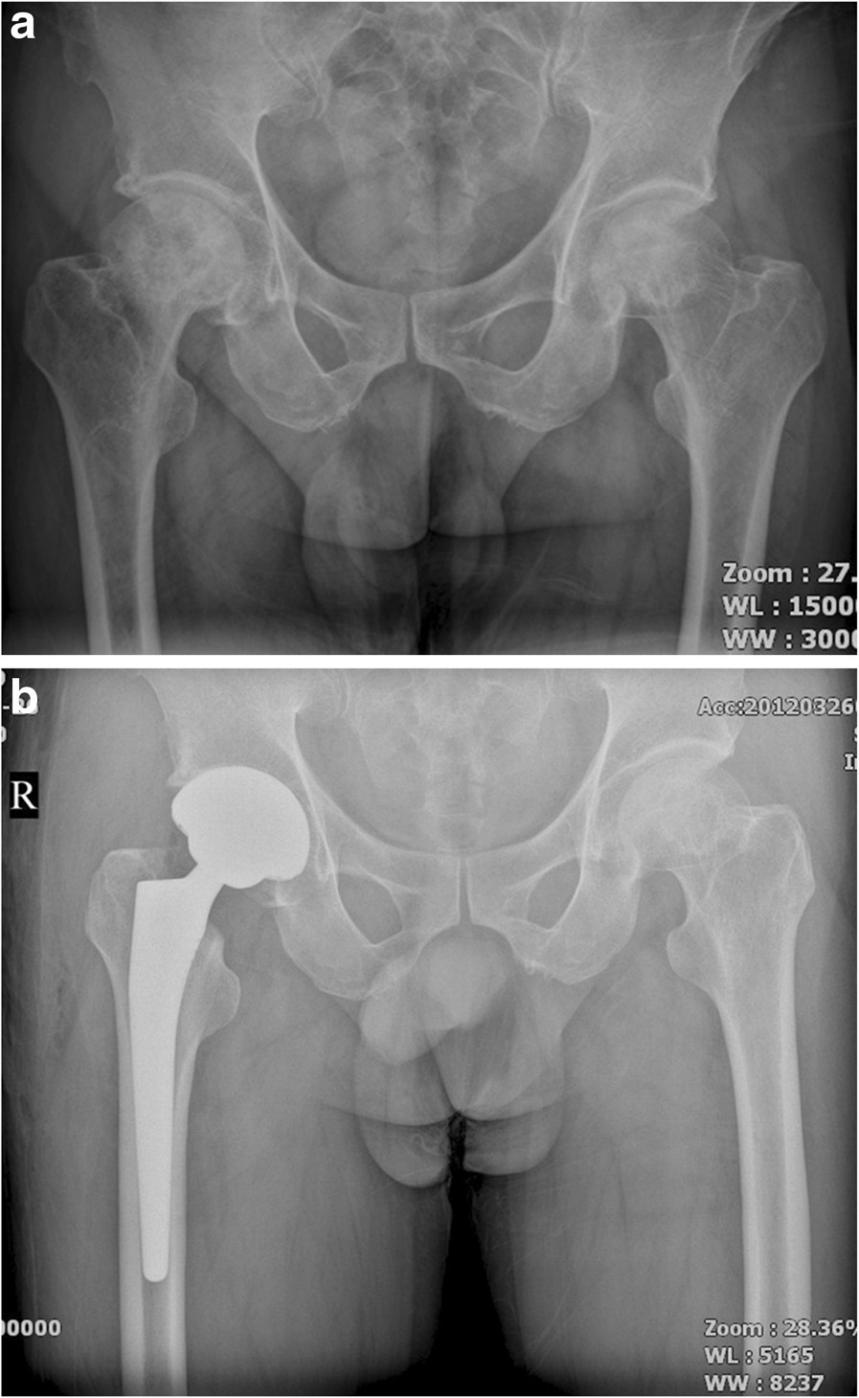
a A preoperative radiograph of the hip of a 73-year-old man who had femoral head osteonecrosis. b A radiograph at 28 months after implantation of conventional cementless femoral stem showed solid fixation in a satisfactory position
